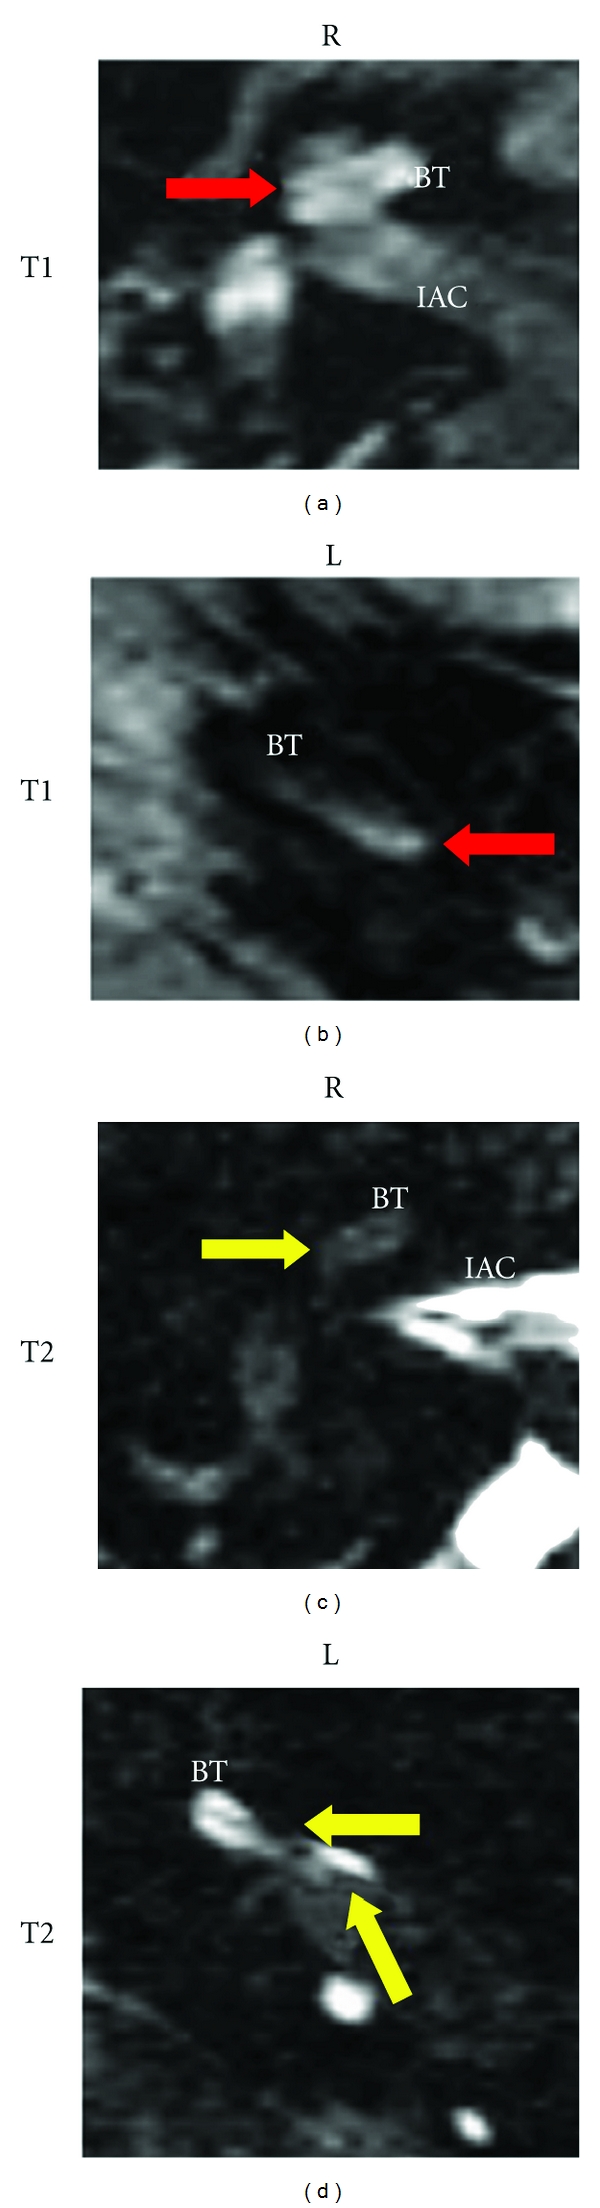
MR images of the right (R) and left (L) inner ears of a patient (case 2) after pneumococcal meningitis. Depicted are the axial T1 weighted MR images with contrast enhancement (T1, top row) and the T2 weighted MR images (T2, bottom row). The patient, a boy aged 7 months, suffered from asymmetric hearing loss after pneumococcal meningitis. Auditory brain stem response (ABR) audiometry showed a deaf ear on the right side and a sloping hearing loss (60 dB at 3 KHz) on the left side. Red arrows show contrast enhancement in the cochlea on the T1 weighted images of both ears ((a) and (b)). The contrast enhancement involves the whole cochlea and vestibulum on the right side, but it is limited to the basal turn (BT) on the left. Yellow arrows show loss of fluid in the cochlea on the T2 weighted images on both sides ((c) and (d)). Whereas on the right side, the loss of fluid involves the complete cochlea and the basal turn is barely visible, the loss of fluid only partially involves the basal turn of the left cochlea. IAC: internal auditory canal.
